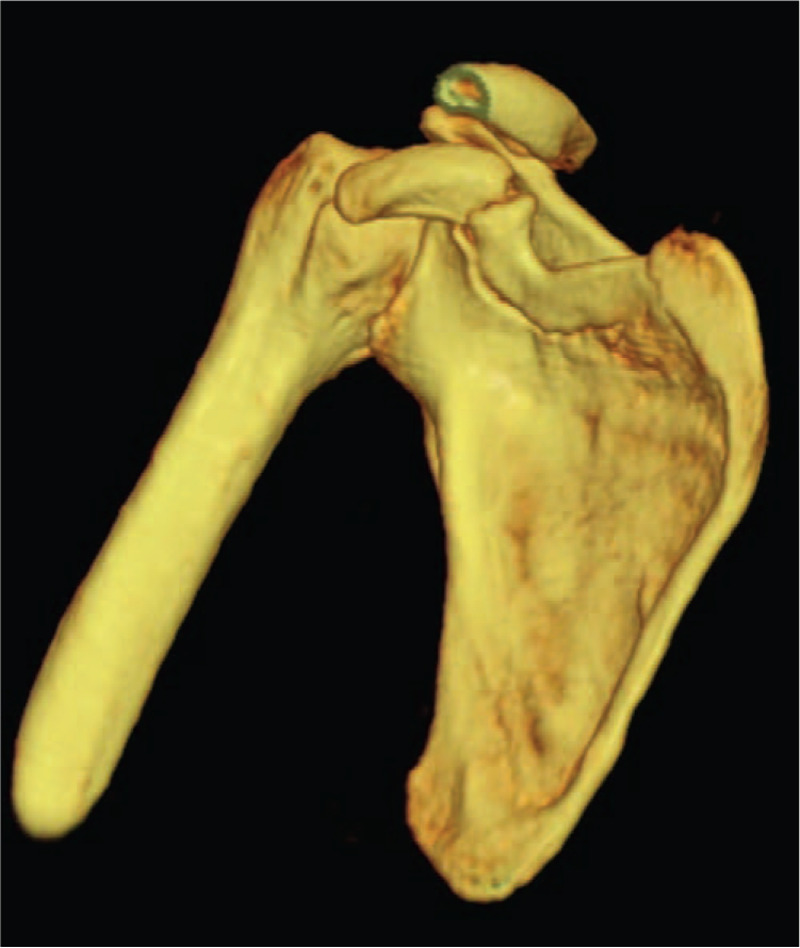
Preoperative 3D-CT showed a displaced fracture of the coracoid process base.
